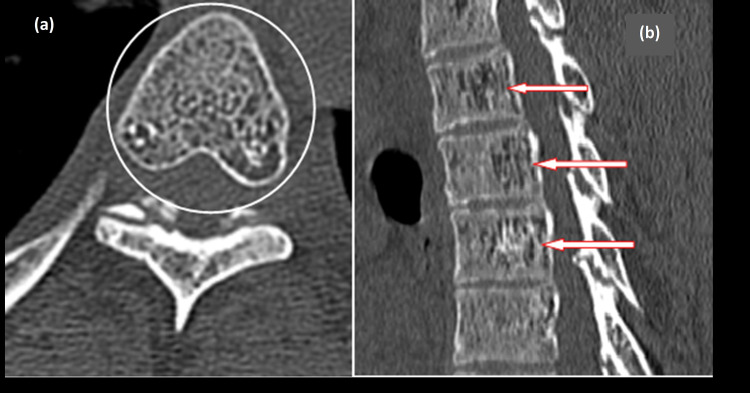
(a) Depicts an axial view of the CT scan showing classical polka dot appearance of the hemangioma, which is occupying most of the vertebral body and extending into both the pedicles and the lamina. (b) Shows a sagittal view of the same CT scan showing the classic "corduroy cloth" or "jail-bar" appearance of the vertebral bodies, highly indicative of a vertebral hemangioma
